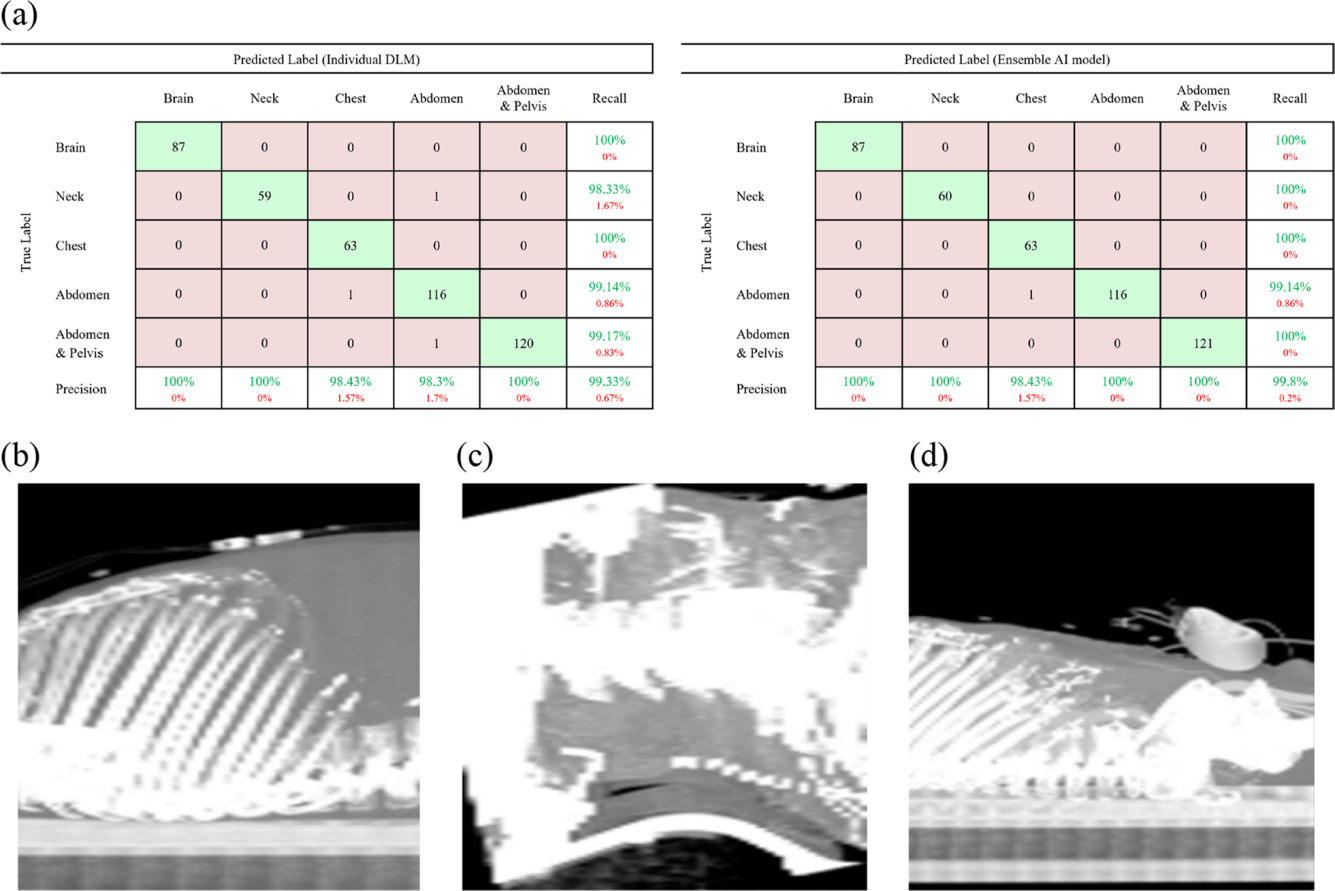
Comparison between the individual DLM and the ensemble AI model for body art classification based on the external dataset. a Confusion matrices of the best performing individual DLM and the ensemble AI model. b Abdominal CT misclassified as chest CT in both the best performing individual DLM and the ensemble AI model. c Neck CT misclassified as abdominal CT from the best performing DLM. d Abdominopelvic CT misclassified as abdominal CT from the best performing DLM
